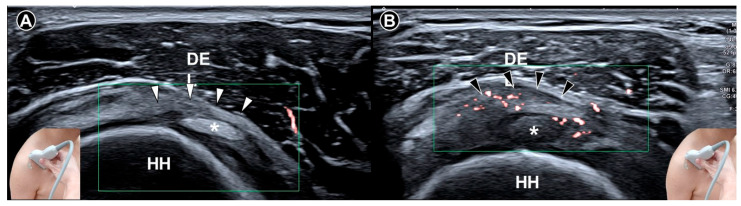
Ultrasound imaging of the rotator cuff interval *(white arrowheads)* in a normal shoulder (A). In contrast, the coracohumeral ligament *(black arrowheads)* in a shoulder with adhesive capsulitis exhibits increased vascularity (B). DEL, deltoid muscle; HH, humeral head; *asterisks*, long head tendon of the biceps brachii.
